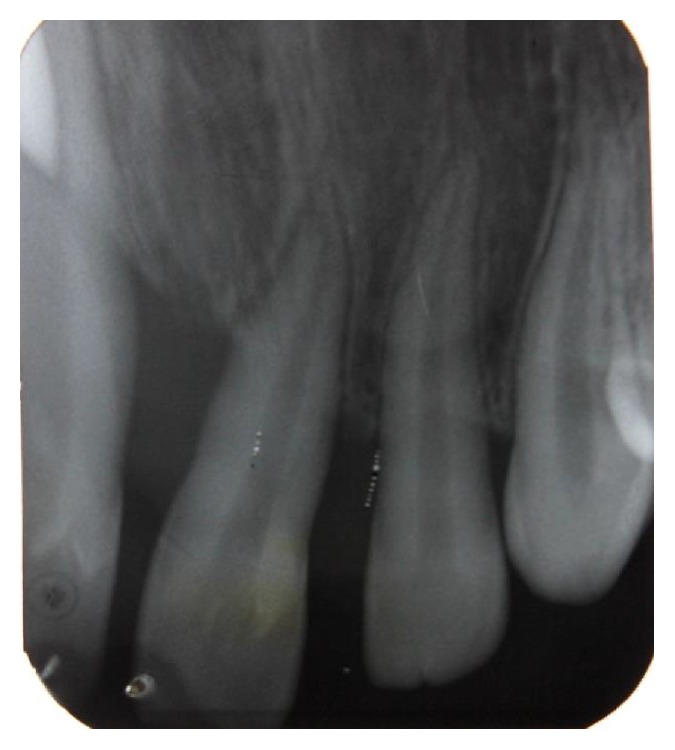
Intraoral periapical radiograph showing bone loss in relation to 11, 12.
